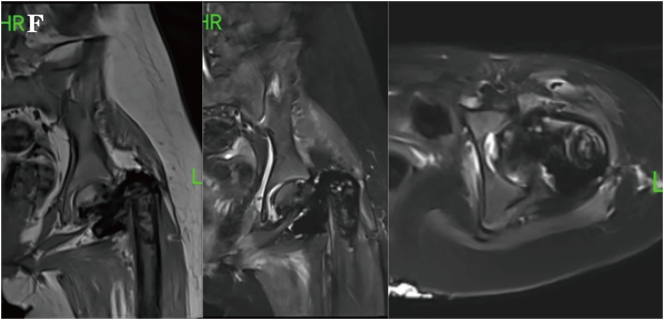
MRI after three cycles of chemotherapy showed a reduction in the size of the mass.
